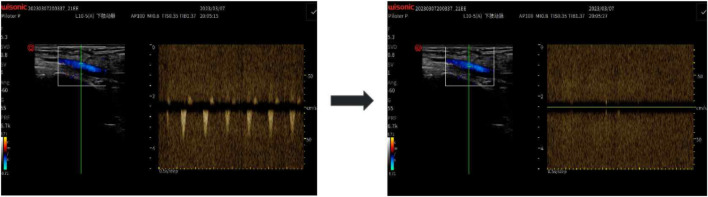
Doppler Ultrasound Measurement of Arterial Occlusion Pressure (AOP): Using a handheld Doppler probe (WISONIC Piloter S), blood flow in the posterior tibial artery was monitored while a thigh cuff was inflated. AOP was defined as the pressure at which the Doppler signal disappeared.
